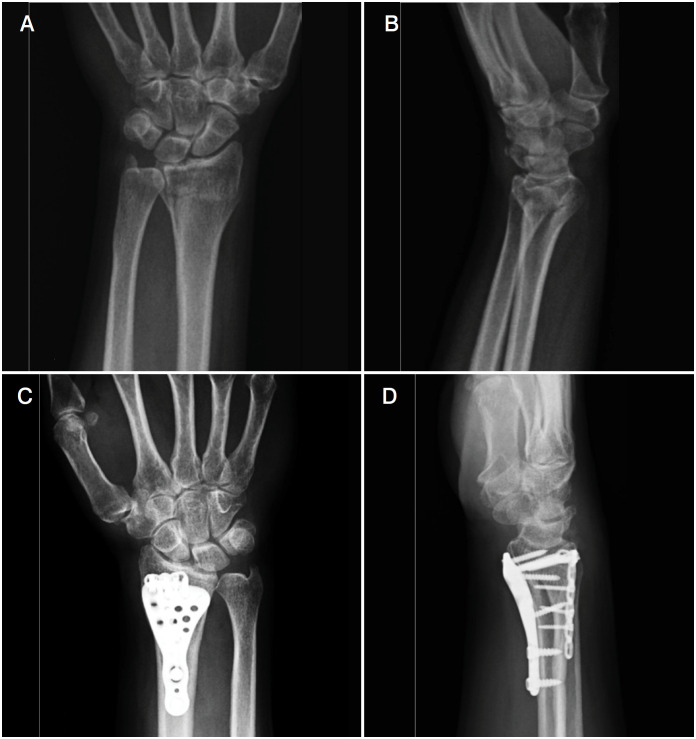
A) Preoperative anteroposterior radiograph showing distal radius fracture, AO 2R3-C3, Melone type 2a. B) Preoperative lateral radiograph showing distal radius fracture, AO 2R3-C3, Melone type 2a. C) X-ray with anteroposterior after fixation with the combined volar-dorsal plates. D) X-ray with lateral after fixation with the combined volar-dorsal plates.
